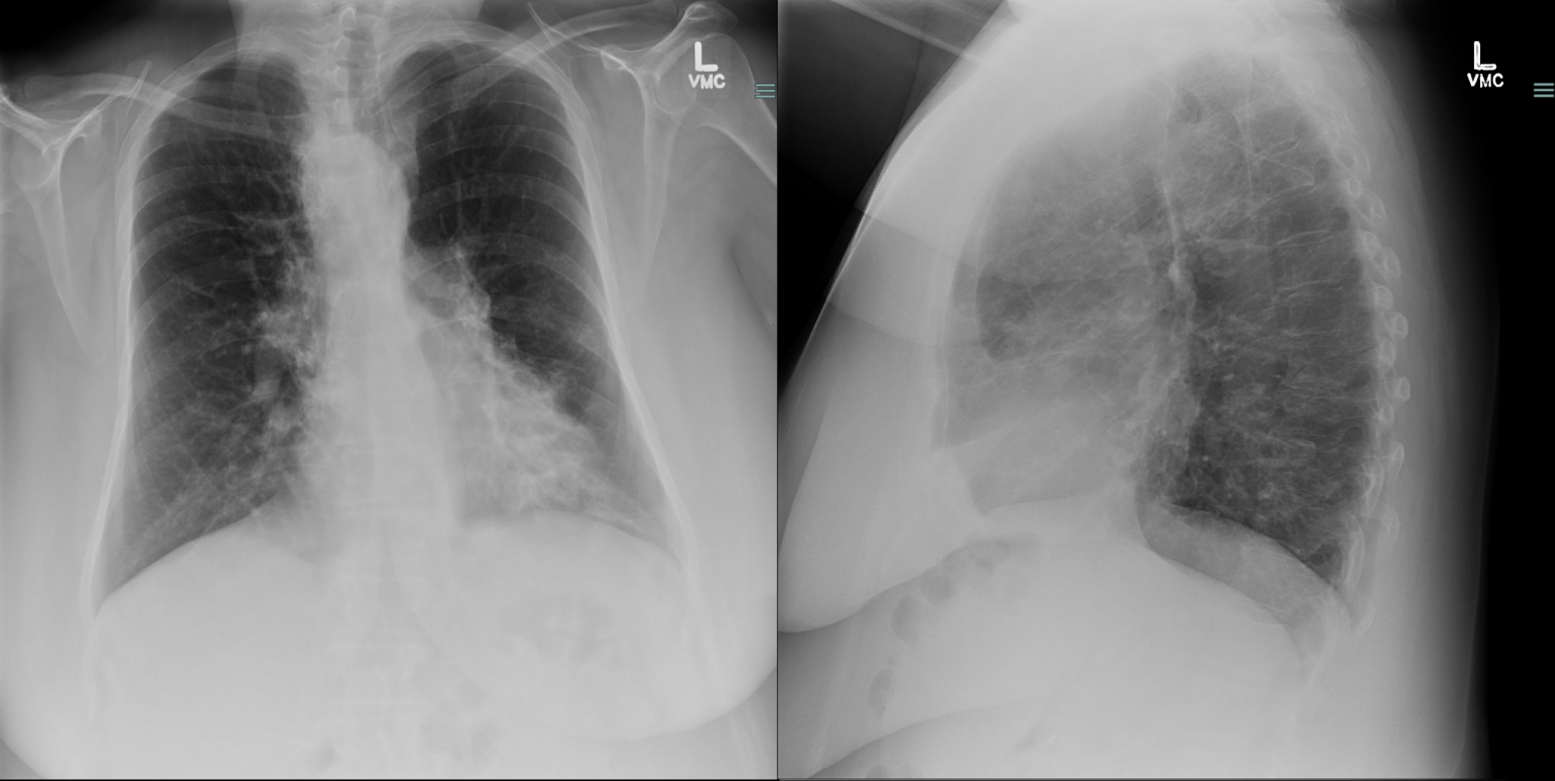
The posteroanterior and lateral chest radiographs demonstrated hyperexpansion of the lungs and bronchiectasis in the right suprahilar region and left lung base, along with presence of a right-sided aortic arch.
